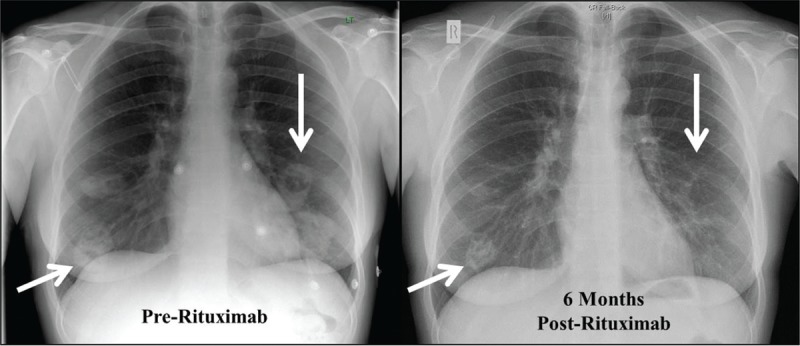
Chest radiographs performed pre-rituximab and after 6 months in Patient 2 are shown. Far left arrows show the largest cavitating pulmonary nodule, which reduced in size from 3.6 to 2.9 cm after treatment. The downward arrows to the right of each CXR show almost complete resolution of a cavitating nodule present at the start of treatment.
